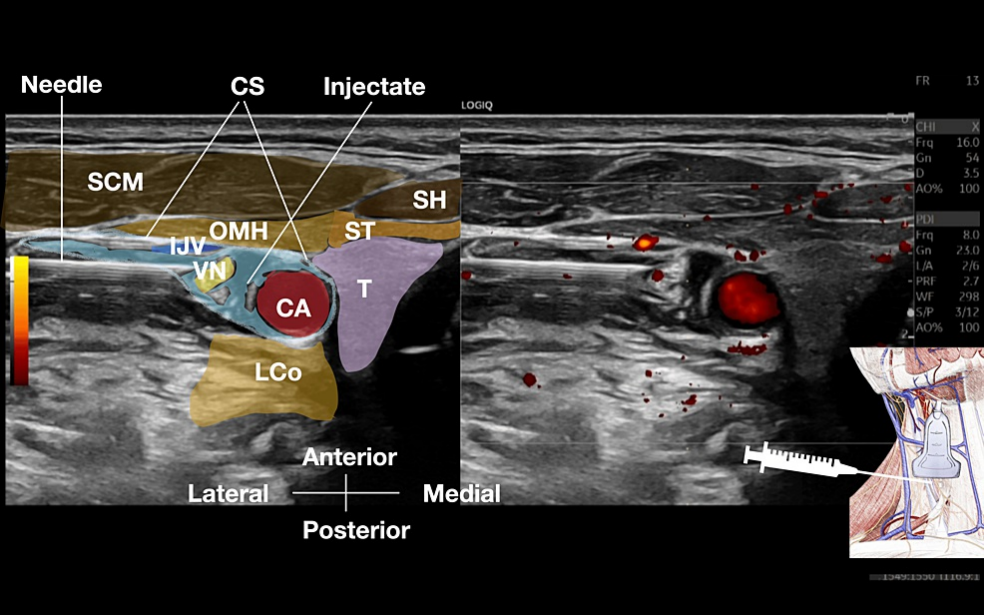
Ultrasound-guided hydrodissection of the vagus nerve within the carotid sheath using 5% dextrose as the injectate. CA, Carotid Artery; CS, Carotid Sheath; IJV, Internal Jugular Vein; LCo, Longus Coli; OMH, Omohyoid; SCM, Sternocleidomastoid; SH, Sternohyoid; ST, Sternothyroid, T, Thyroid, VN, Vagus Nerve
